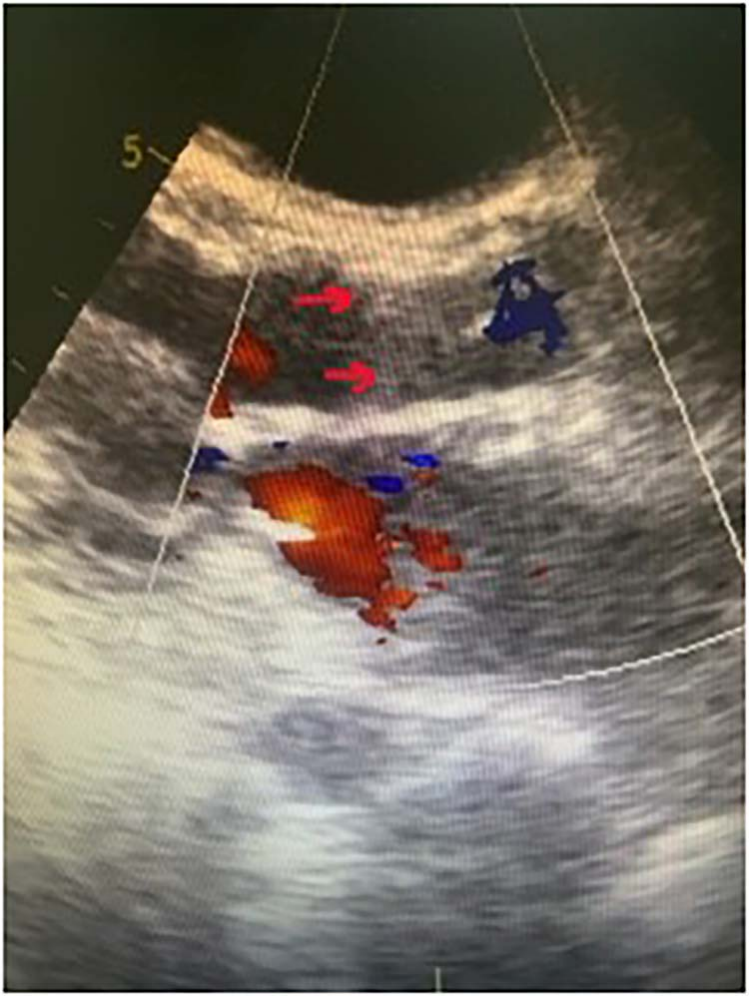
Doppler echocardiography after TAVI procedure shows valve in place (red arrows) and no residual regurgitation.
